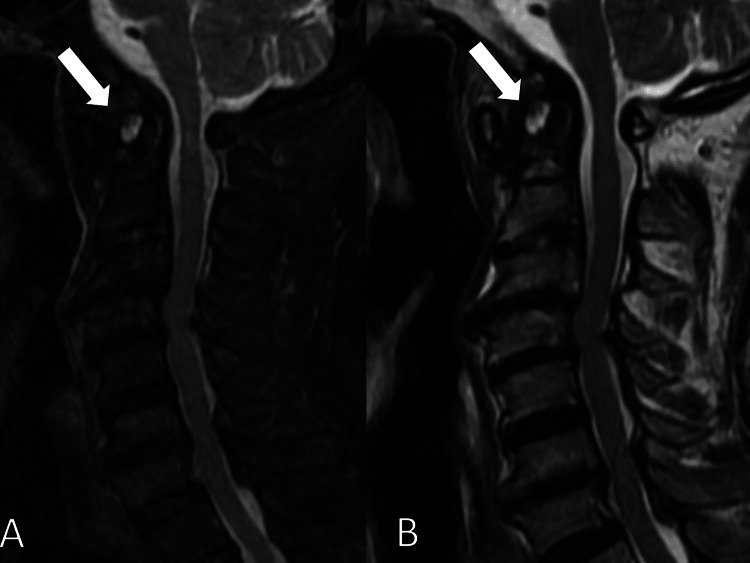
MRI of high signal areas of dens, showing the inflammation of the dens axis (white arrows) (A) T2 enhanced; (B) short-tau inversion recovery
